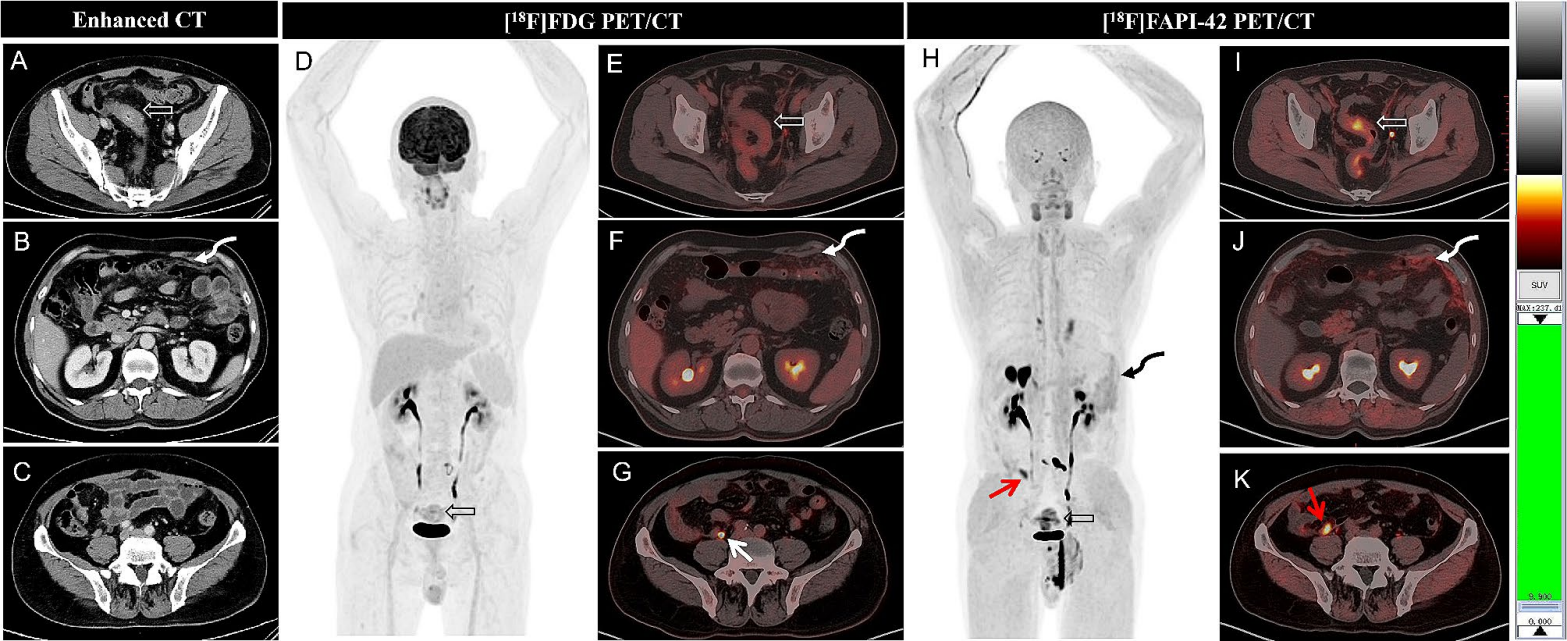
A 60-year-old man complained increased number of defecations, tumor markers of CA724 and CEA. The axial CE-CT showed thickening of the sigmoid colon wall (A, hollow arrow) and peritoneum in the left upper abdomen (B, bent arrow), suggested sigmoid colon cancer with peritoneal metastasis. The maximal intensity projection (MIP) of ^18^F-FDG PET/ CT showed mild ^18^F-FDG uptake in the pelvis (D, hollow arrow). The axial fused image PET/CT showed mild ^18^F-FDG uptake in the sigmoid colon (SUVmax, 3.0, E, hollow arrow) and omentum majus (SUVmax,1.6, F, bent arrow). The focus next to the appendix was physiologic uptake of ureter (G, white arrow). However, the MIP of ^18^F-FAPI-42 PET/ CT showed medium ^18^F-FAPI uptake in the lower right abdomen (H, red arrow), upper left abdomen (H, bent arrow) and sigmoid (H, hollow arrow). The axial fused image PET/CT showed moderate ^18^F-FAPI in the sigmoid (SUVmax, 5.4, I, hollow arrow) and omentum majus (SUVmax, 5.0, J, bent arrow), otherwise, the ^18^F-FAPI was also taken up by the lesions obviously in the appendix (SUVmax, 6.2, K, red arrow). Thus, the patient was diagnosed with appendiceal neoplasms and peritoneal metastasis which infiltrated the sigmoid colon. Then the patient underwent sigmoid puncture biopsy, which was diagnosed with goblet cell adenocarcinoma originating from the appendix. ^18^F-FAPI-42 PET/ CT was more sensitive to detect the primary tumor than ^18^F-FDG PET/ CT and CE-CT, although it did not change the clinical treatment plan
